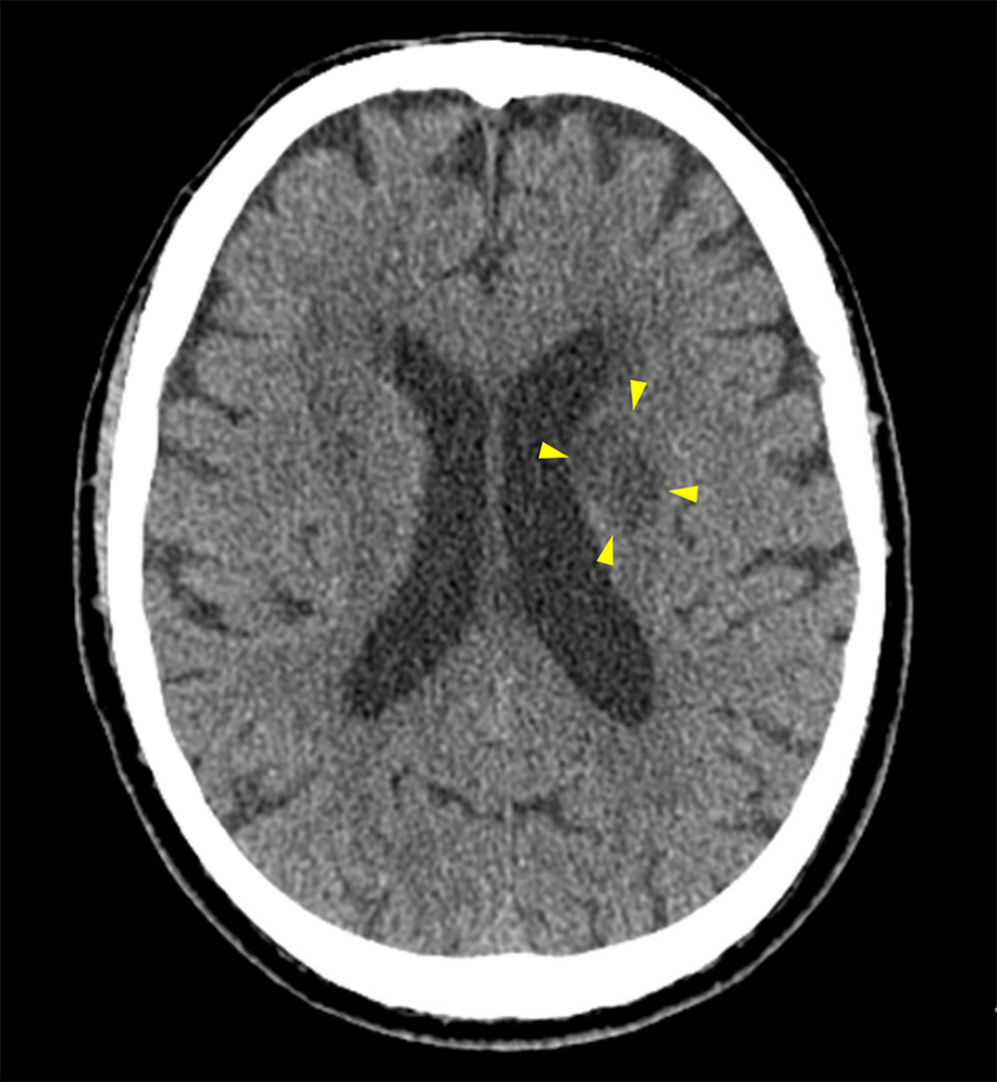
A computed tomography scan on day 5 revealed a low‐density area from the left corona radiata to the basal ganglia, and the patient was diagnosed with acute cerebral infarction.
